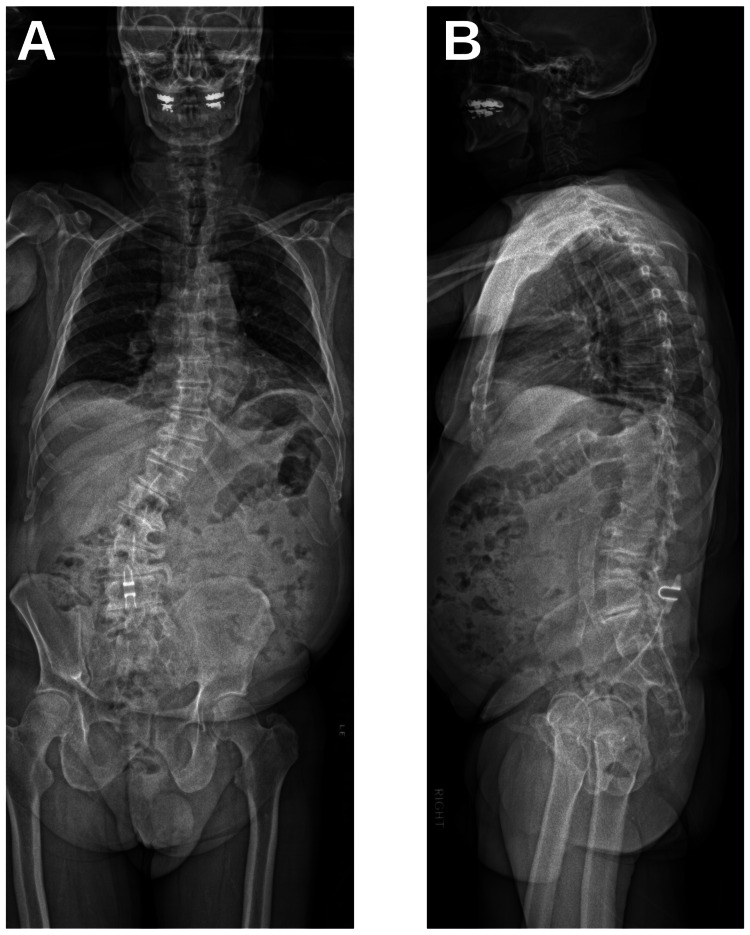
Pre-Operative Radiography Pre-operative coronal (A) and sagittal (B) radiography showing significant scoliotic deformity.
